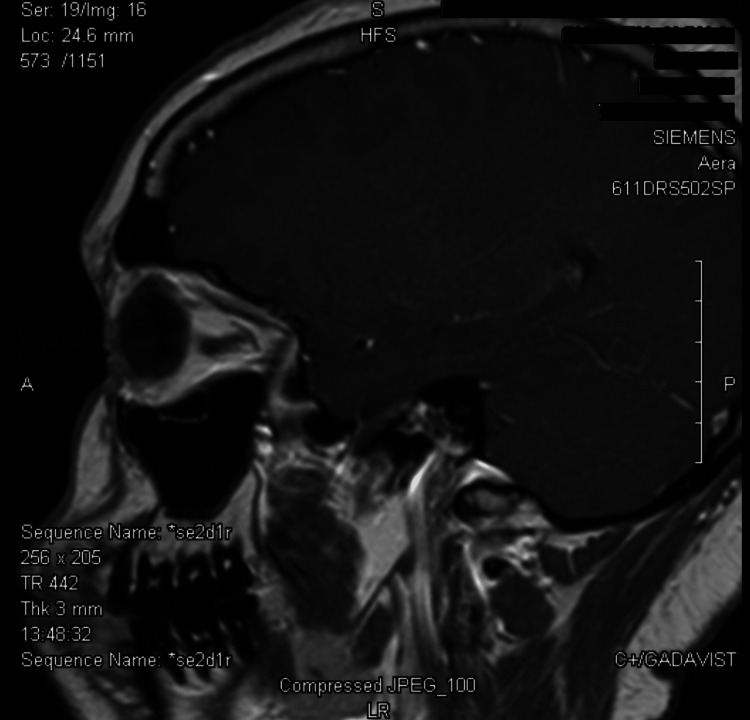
Pituitary MRI with contrast demonstrating a normal-sized gland with homogeneous enhancement and no evidence of adenoma.
